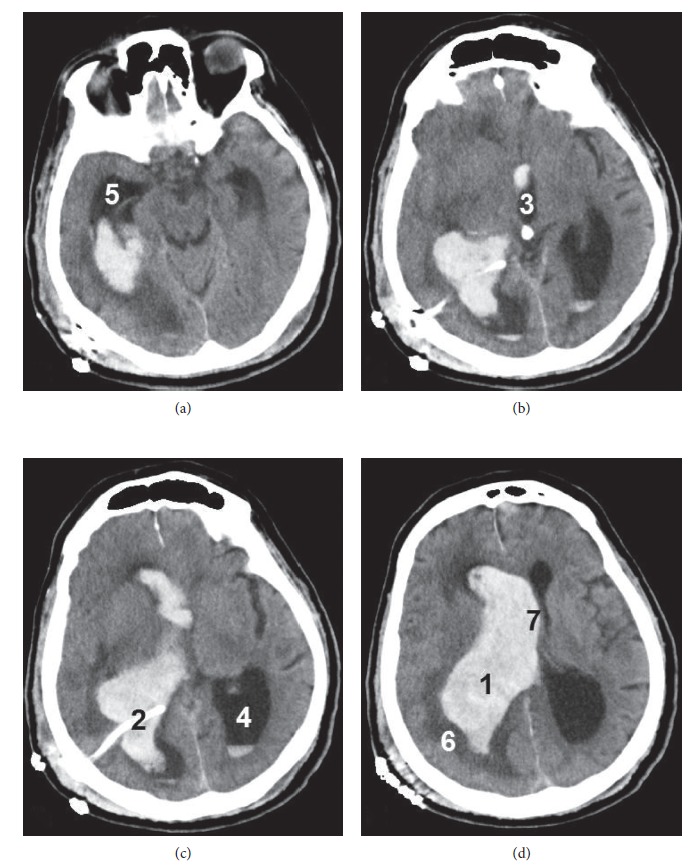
The second postoperative cerebral CT scan of our patient (fifth postoperative day). (a), (b), (c), (d) Different views (from inferior to superior). Note the large IVH within the right lateral ventricle (1), around the ventricular catheter (2), with extension into the third (3) and left lateral (4) ventricles. The right lateral ventricle is dilated (5), with apparent periventricular edema (6) and midline shift (7).
